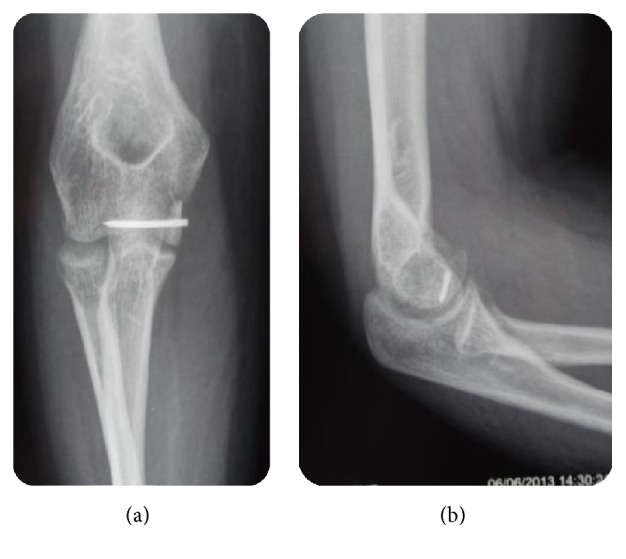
Immediate postoperative radiographs after fixation (a, b) anteroposterior and lateral views of the elbow.
